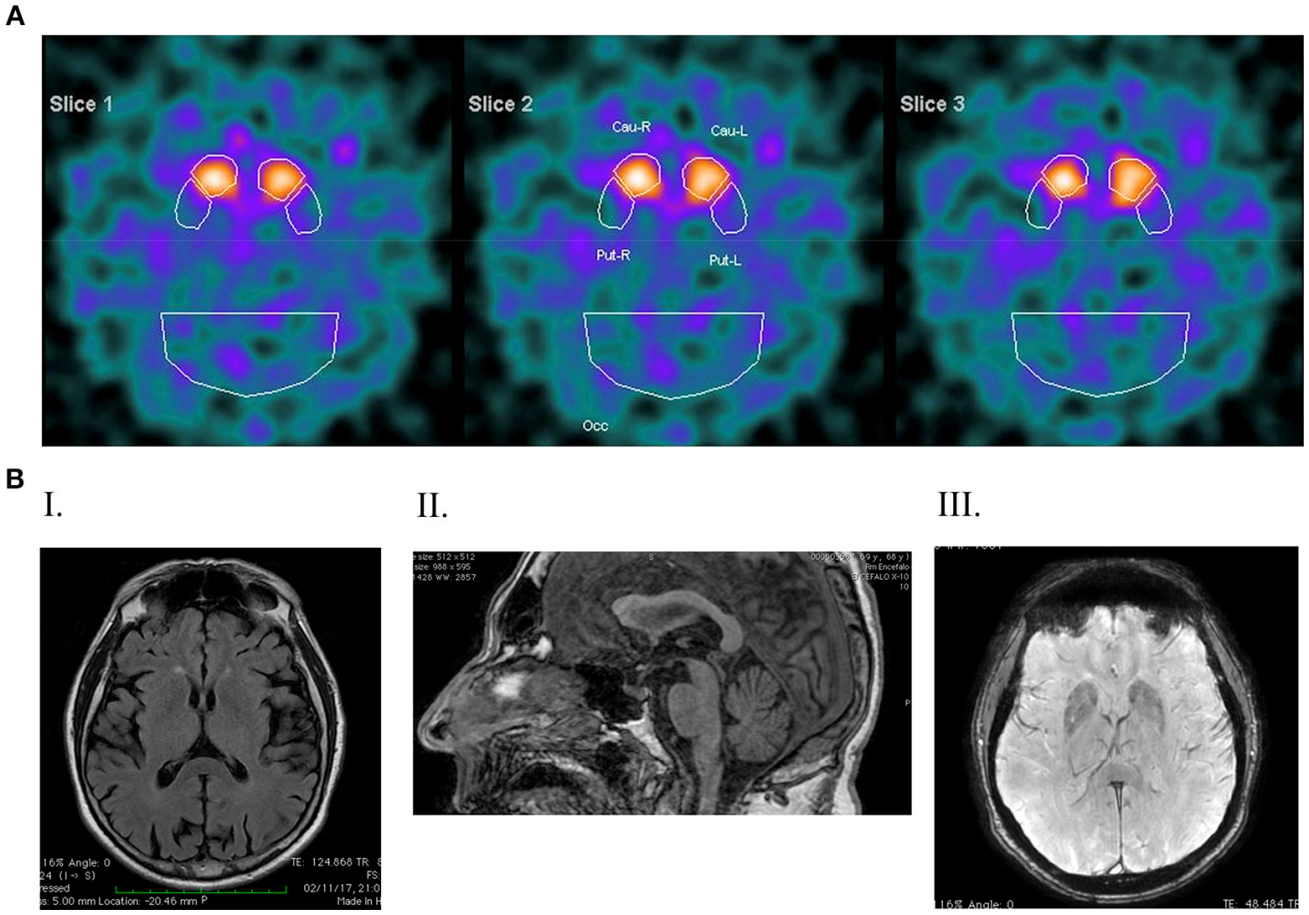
DaTSCAN and Magnetic Resonance Imaging (1,5 Tesla). (A) DATSCAN image showing a reduced tracer uptake in both striatal regions; (B) magnetic resonance imaging. (I) T2 FLAIR shows post-central atrophy and bilateral temporo-insular atrophy; (II) SWAN shows a reduced thickness of the mesencephalic tagmen; (III) T1 FSPGR shows signs of iron deposition in the globus pallidum and in both putamen.
